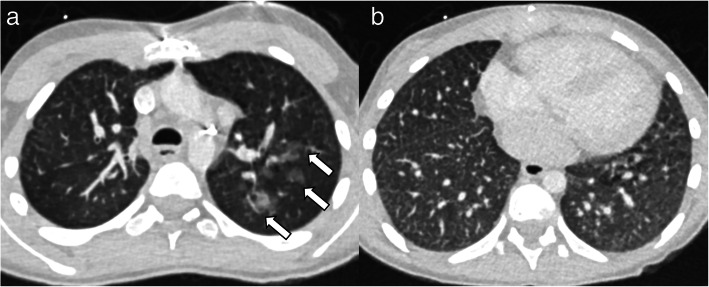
Chest CT findings in a 13-year-old female with trisomy 21 (Patient 4). Axial CT images through the upper (a) demonstrate ill-defined nodular ground glass opacities in the left upper lobe (arrows). Axial CT image through the lower chest (b) demonstrates innumerable tiny centrilobular nodules and septal thickening bilaterally
